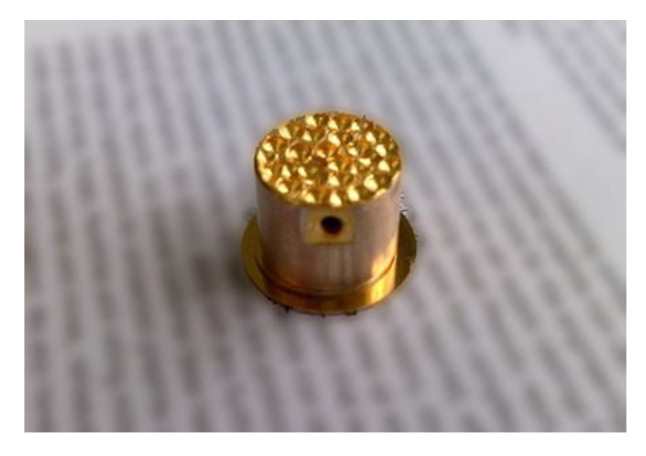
Ultrasonic transducer with a graphite bronze backing on the top.
